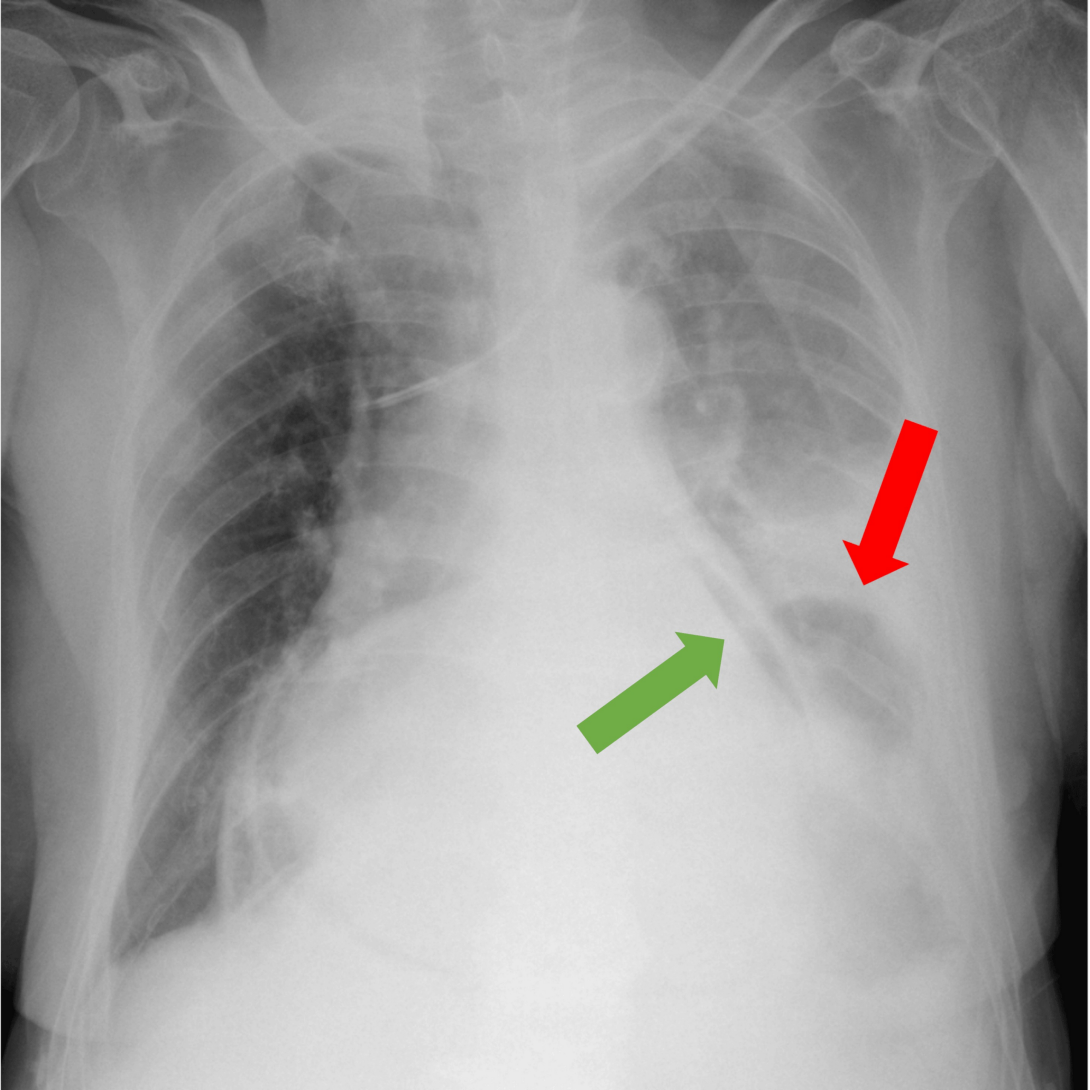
Chest native X-ray in anteroposterior projection The green arrow shows gas in the pericardial area caused by the fistula with gastroenteric communication. The red arrow shows the gastric gas in the chest cavity caused by the giant hiatal hernia.
